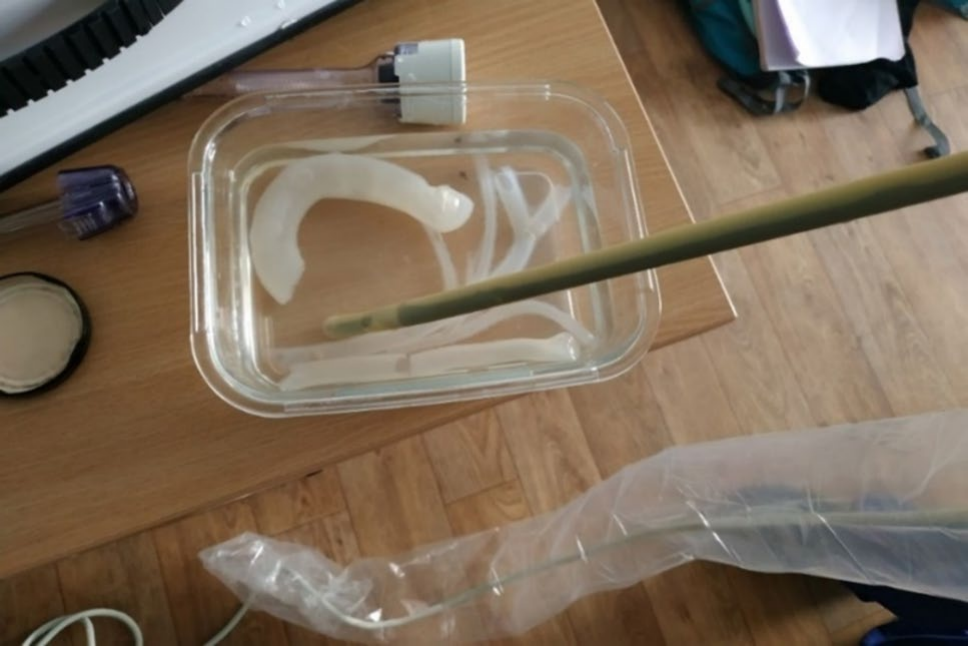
Recording of the ultrasound images on the agar model using a laparoscopic ultrasound probe
